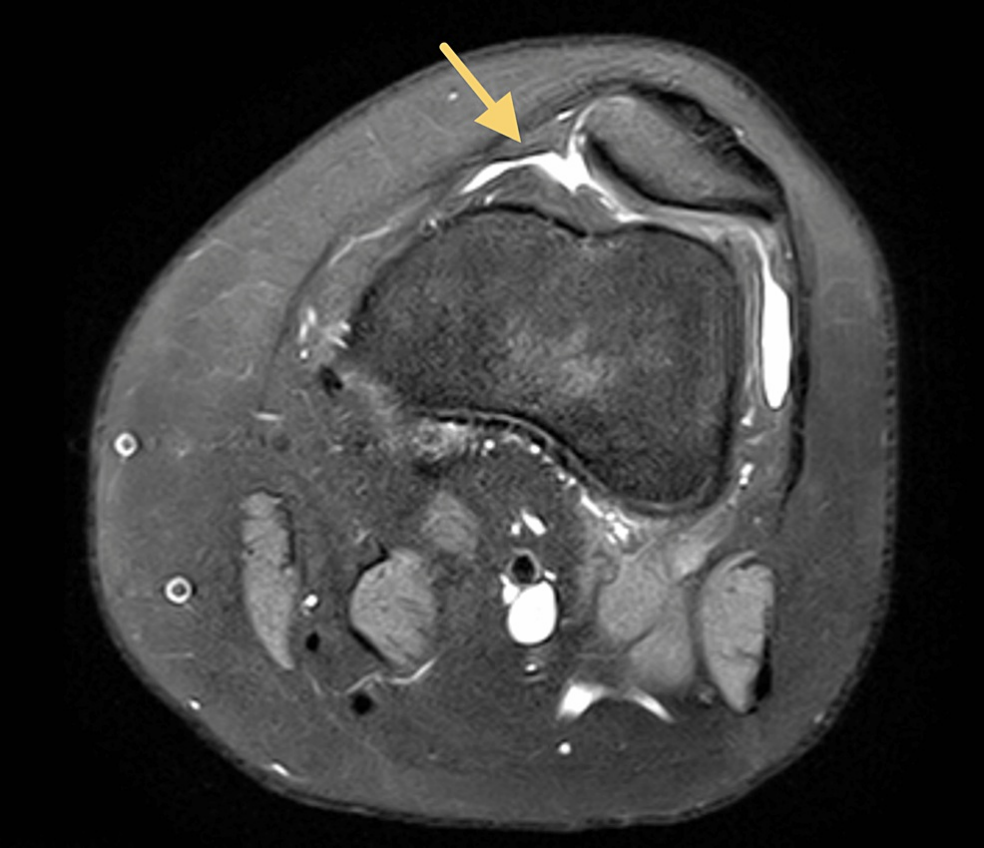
MRI of the knee for the second patient, which demonstrates trochlear dysplasia with a shallow groove, patellar tilt, and lack of patellar containment with the patella overhanging the lateral facet of the trochlea. The arrow indicates the region of trochlear dysplasia with a shallow groove, patellar tilt, and lack of patellar containment.
